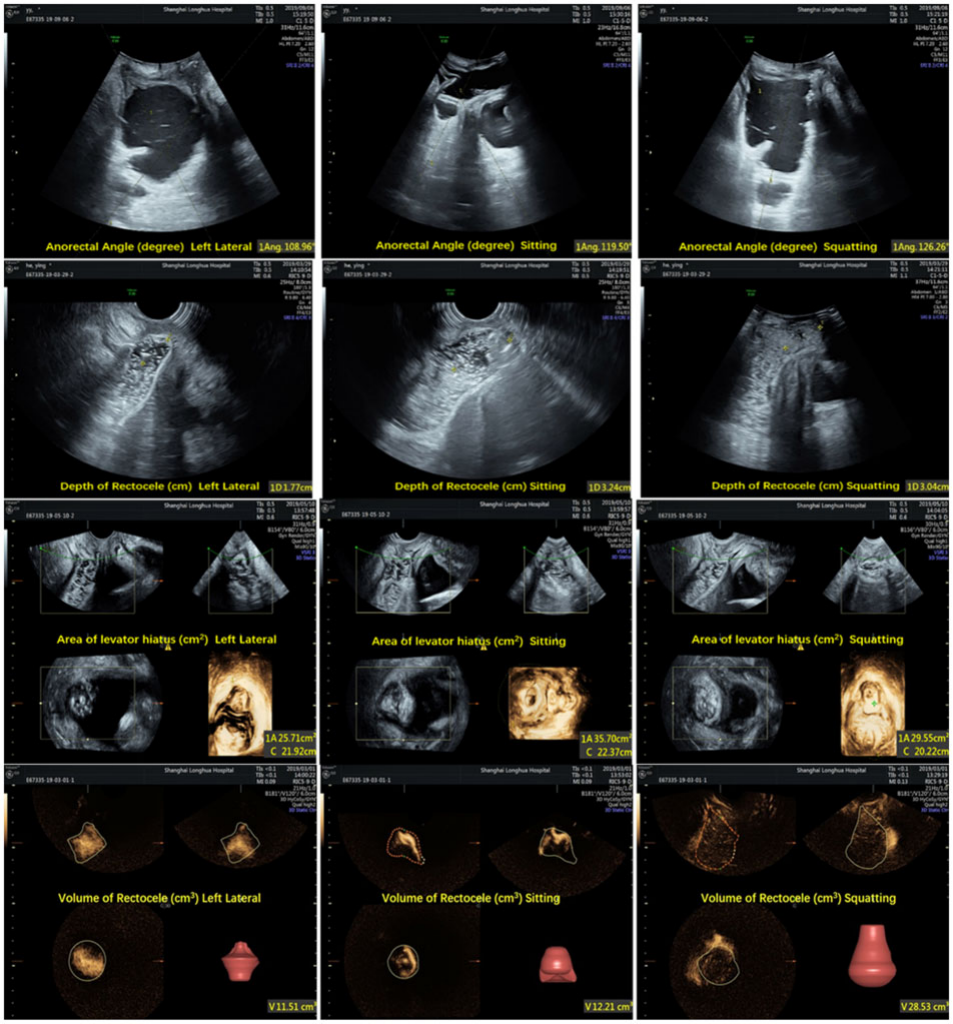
Anorectal angle, depth of rectocele, area of levator hiatus, and volume of rectocele (at maximal Valsalva) by transperineal ultrasonography in three positions
